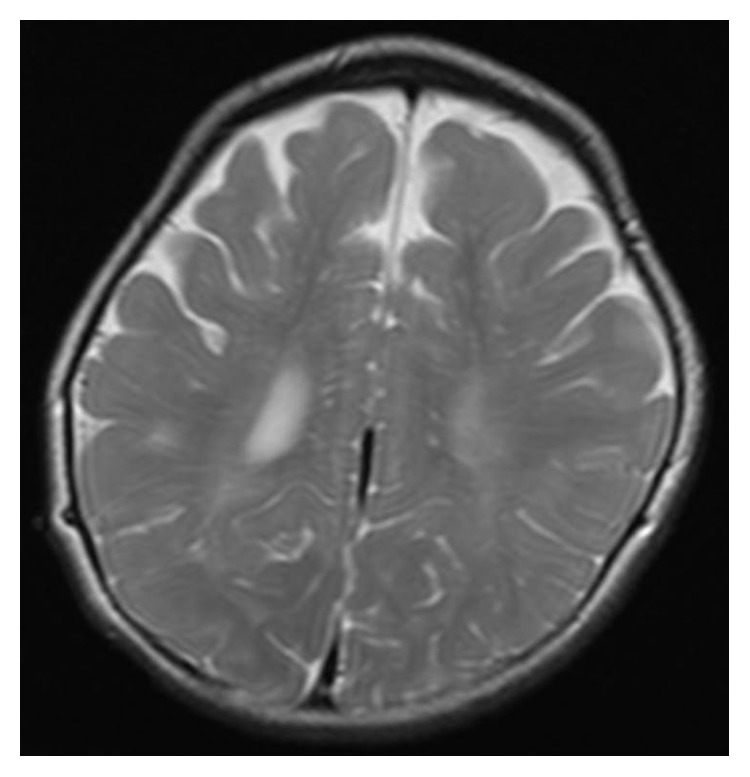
Head magnetic resonance image revealing an enlarged Virchow-Robin space.
